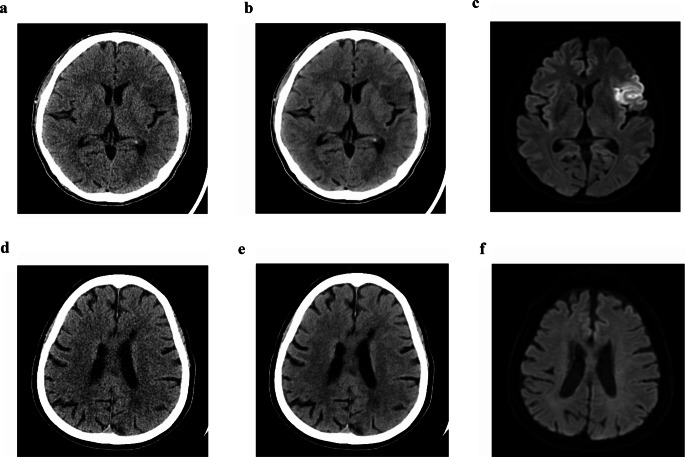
Representative cases. Case 1: 60-year-old male with acute left middle cerebral artery superior division infarction. Iterative reconstruction (IR) (a) and deep-learning reconstruction (DLR) (b) images are shown pairwise with corresponding diffusion-weighted imaging (DWI) (c) acquired within 30 min of CT. Two resident readers scored IR as 1 (no acute lesion), DLR as 3 and 4 (intermediate, high probability). Neuroradiologists scored IR 3, 4 and DLR 4. Case 2: 79-year-old female presented with dysarthria (onset 4.5 h). IR (d) and DLR (e) images are shown; DWI obtained within 30 min (f) and follow-up DWI at 4 days (not shown) revealed no acute ischemia-infarction lesion. All readers scored IR as 1 (no acute lesion). DLR scores were higher: one neuroradiologist scored 4 (high probability), others scored 3 (intermediate probability), likely due to accentuated chronic ischemic changes on DLR
